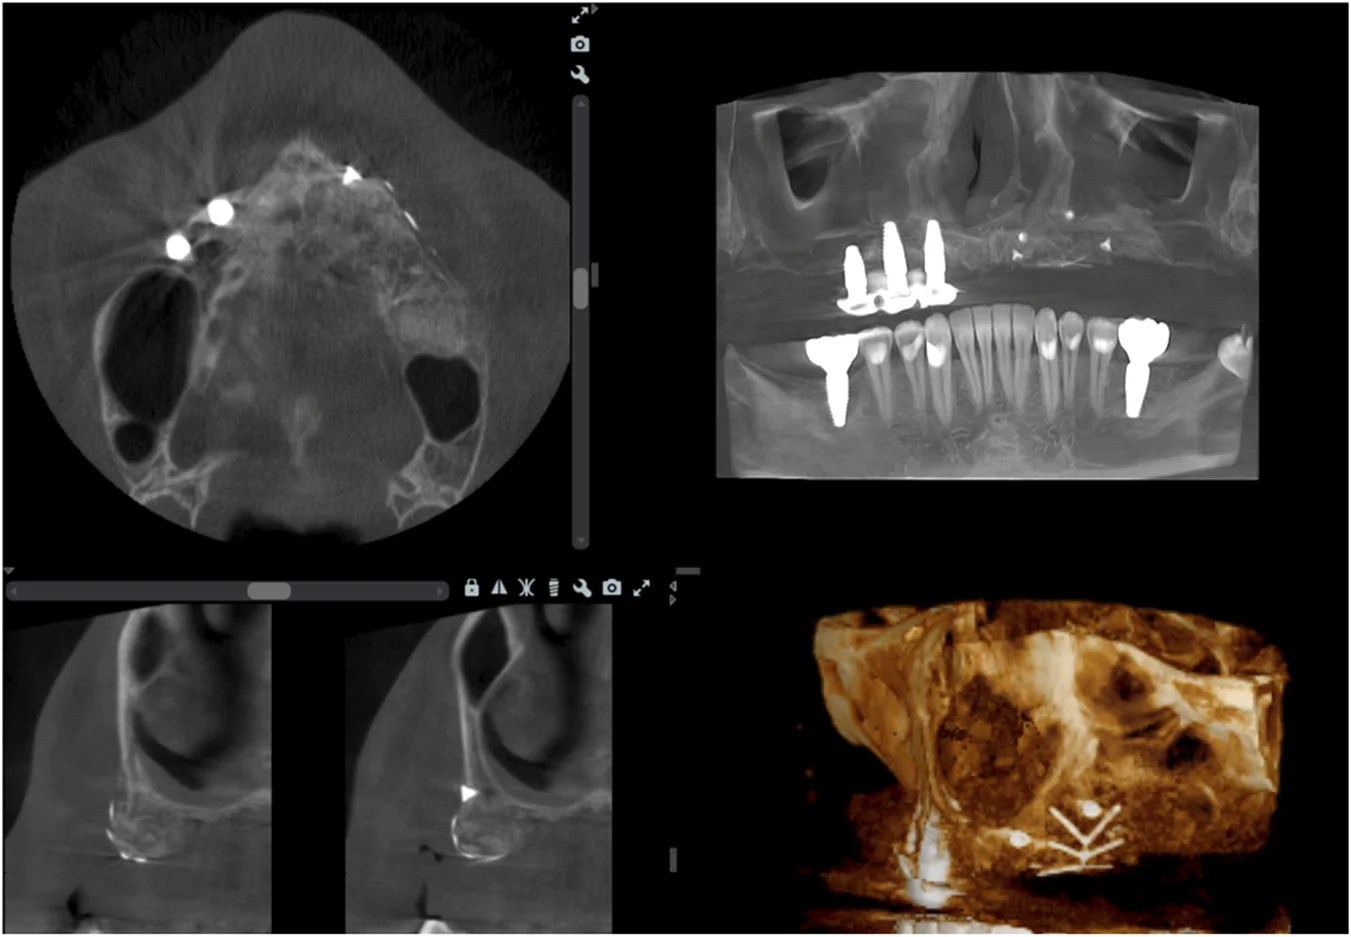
Case 2: 6 months post-grafting. CBCT image and reconstruction showing restoration of alveolar ridge height and width at 6 months post-grafting. Top left: CBCT axial view. Bottom left: CBCT coronal view. Top right: panoramic X-ray. Bottom right: 3D volumetric CBCT reconstruction.
